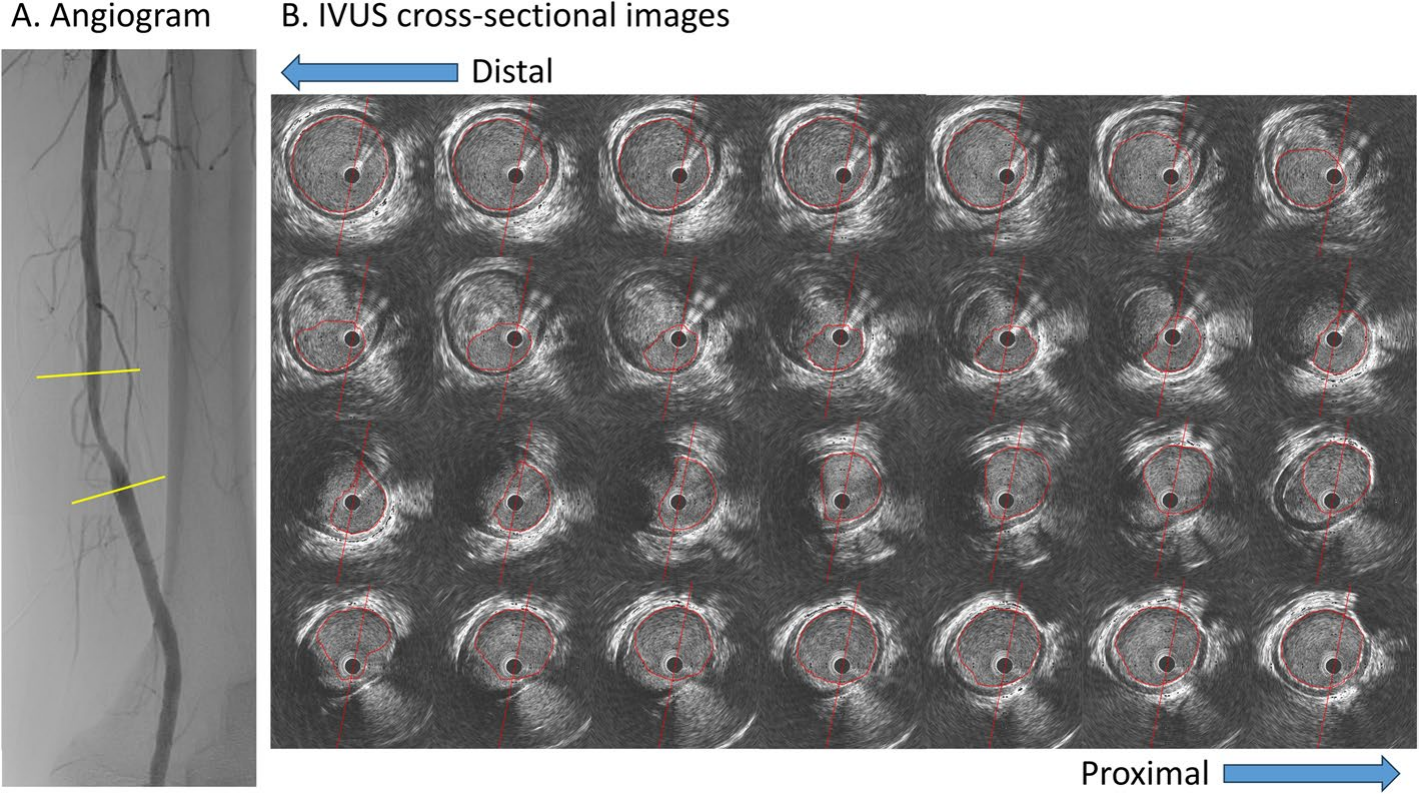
Representative case Luminal surfaces of all cross-sectional IVUS images were traced automatically and corrected manually through the entire lesion. The analysis was performed at 0.167-mm intervals. In this case, a total of 278 slices were analyzed, and 1 out of every 10 images are presented in this figure. Abbreviations: IVUS, intravascular ultrasound.
